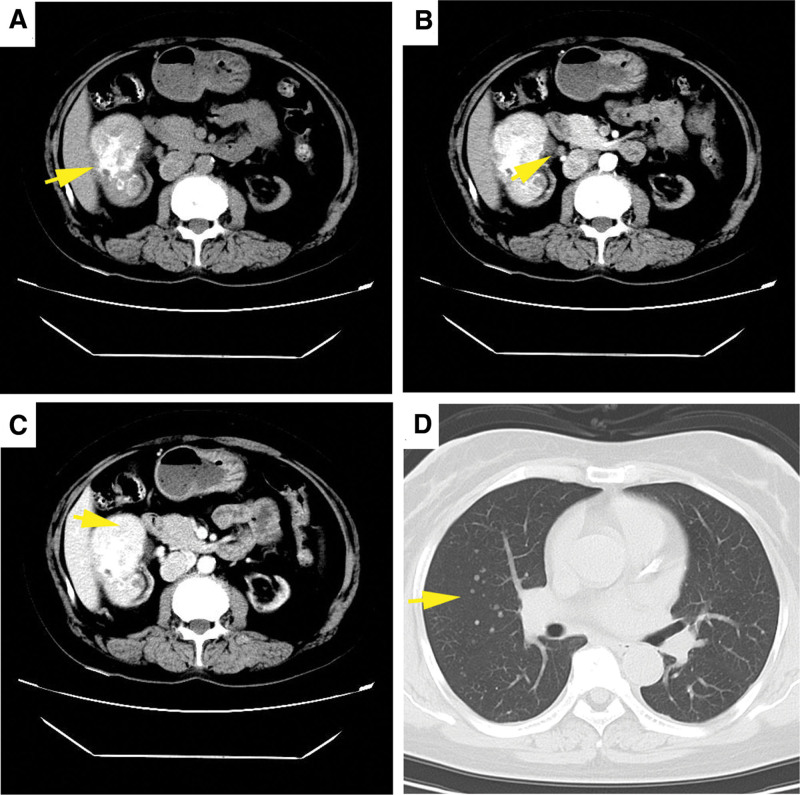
Computed tomography (CT) images of the patient. On the contrast-enhanced CT (CECT), a heterogeneous ill-defined solid-cystic mass was revealed in the right kidney with multiple circular and nodular calcification (A). On the CECT, the parenchyma of the tumor was continuously enhanced, and enlarged lymph node shadows can be seen at the renal hilum, but the cystic component was not enhanced (B and C). The chest CT revealed more new and larger pulmonary nodules (D).
